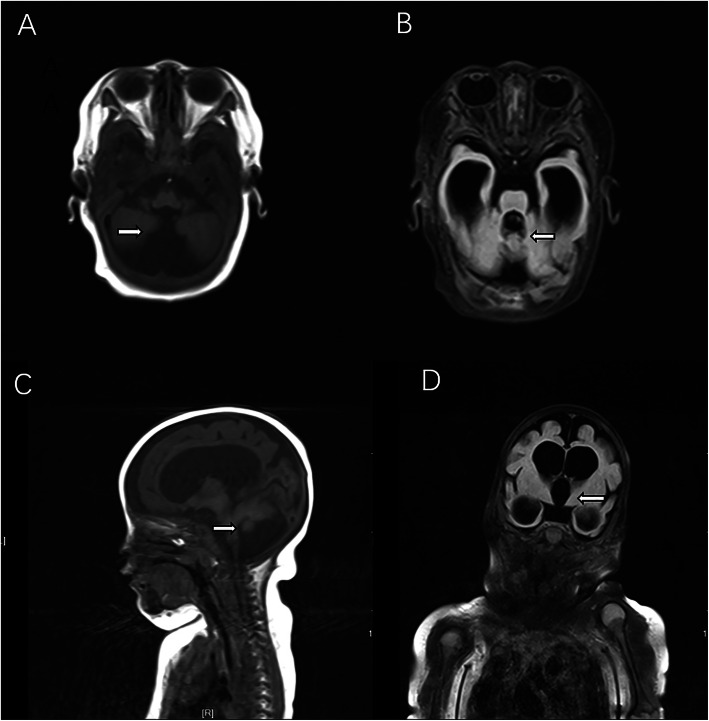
Magnetic resonance imaging of the brain demonstrated cerebellar vermis hypoplasia, enlargement of the fourth ventricle and supratentorial hydrocephalus. Axial T1 weighted imaging (A), Axial T2 weighted imaging with fat and fluid suppression (B), Sagittal T1-weighted imaging (C), Coronal T2 weighted imaging with fat and fluid suppression (D)
